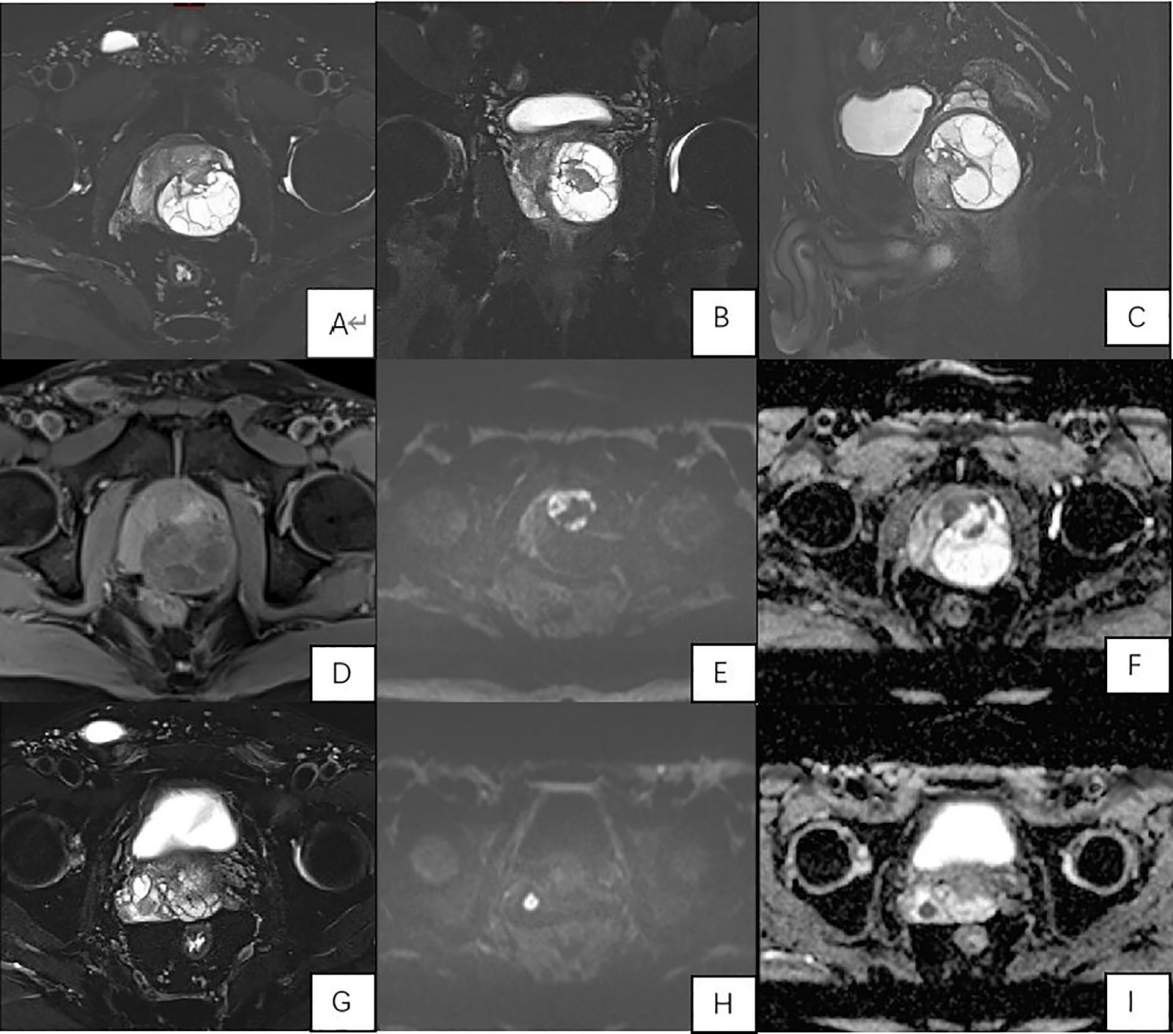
Multiparametric MRI of the Prostate. (A-C) (T2-weighted images): Axial (A), coronal (B), and sagittal (C) views show a large, encapsulated cystic-solid mass. The cystic component is T2-hyperintense with internal septations. The solid component is T2-hypointense; (D) (T1-weighted image): The solid component is iso-intense; (E) (DWI, b=2000): The solid component and the seminal vesicle nodule show high signal, indicating restricted diffusion; (F) (ADC map): Corresponding low signal in the solid component and seminal vesicle nodule confirms restriction; (G-I) (Seminal Vesicle Metastasis): T2-weighted image (G), DWI (H), and ADC map (I) clearly depict the involvement nodule in the right seminal vesicle.
